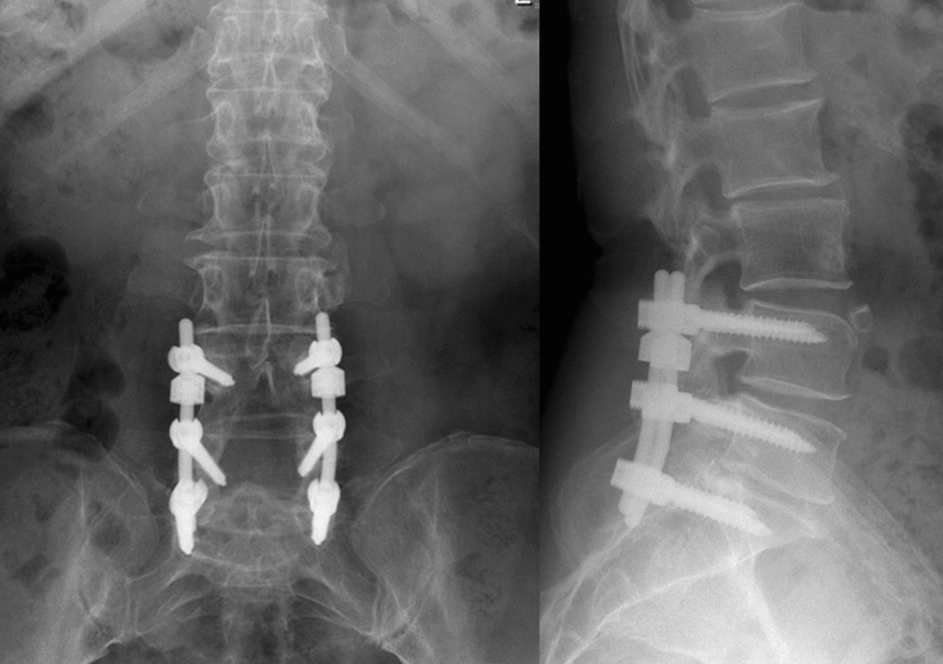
A 53-year-old female complained about his low back pain and intermittent claudication. Pre-operation X-ray indicated mild lumbar spondylolisthesis. She received decompression and Isobar TTL Hybrid surgery
